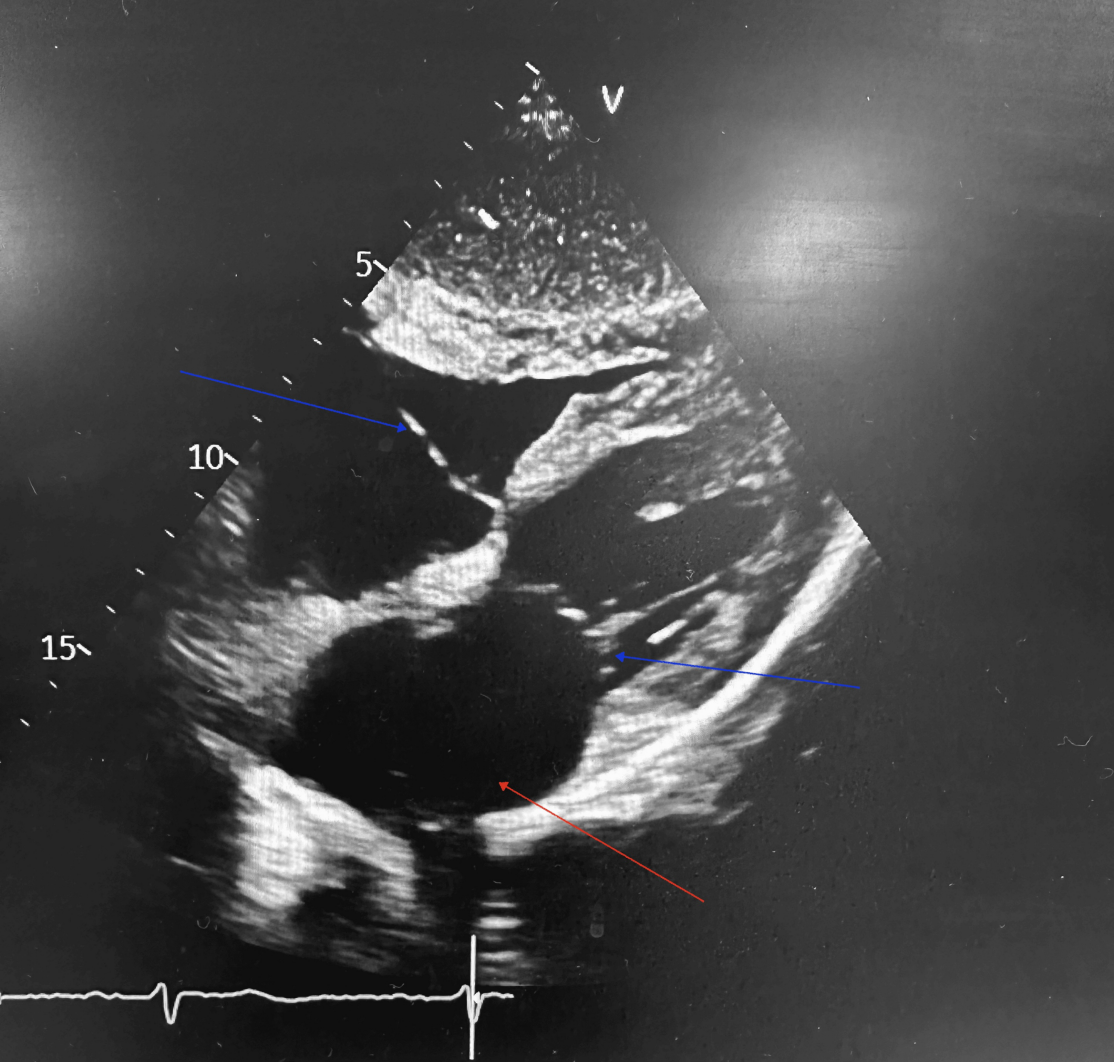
Echocardiogram showing mild left atrial dilatation (red arrow) and clear valves with no vegetations (blue arrows)
